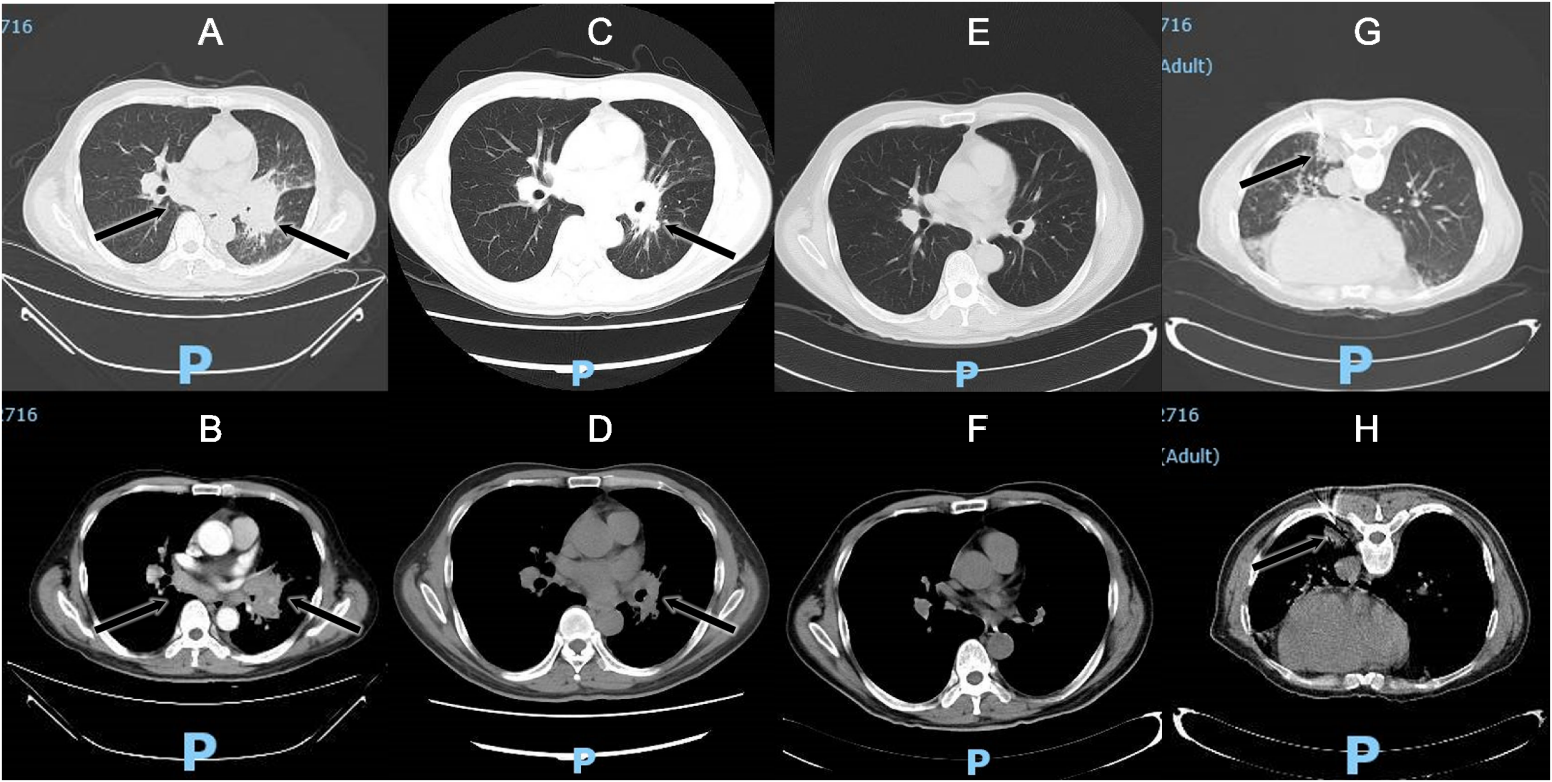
Chest CT scan: subcarinal lymphadenopathy, irregular soft tissue mass in the left hilum and uneven enhancement on the enhanced scan (A) Lung window; (B) Enhanced scanning); Lesions were significantly absorbed on chest CT scans after 16 days of voriconazole treatment (C) Lung window; (D) Mediastinal window); Lesions disappeared on chest CT scans after 6 months of voriconazole treatment (E) Lung window; (F) Mediastinal window); CT-guided percutaneous puncture of the left lower lung biopsy (G) Lung window; (H) Mediastinal window).
